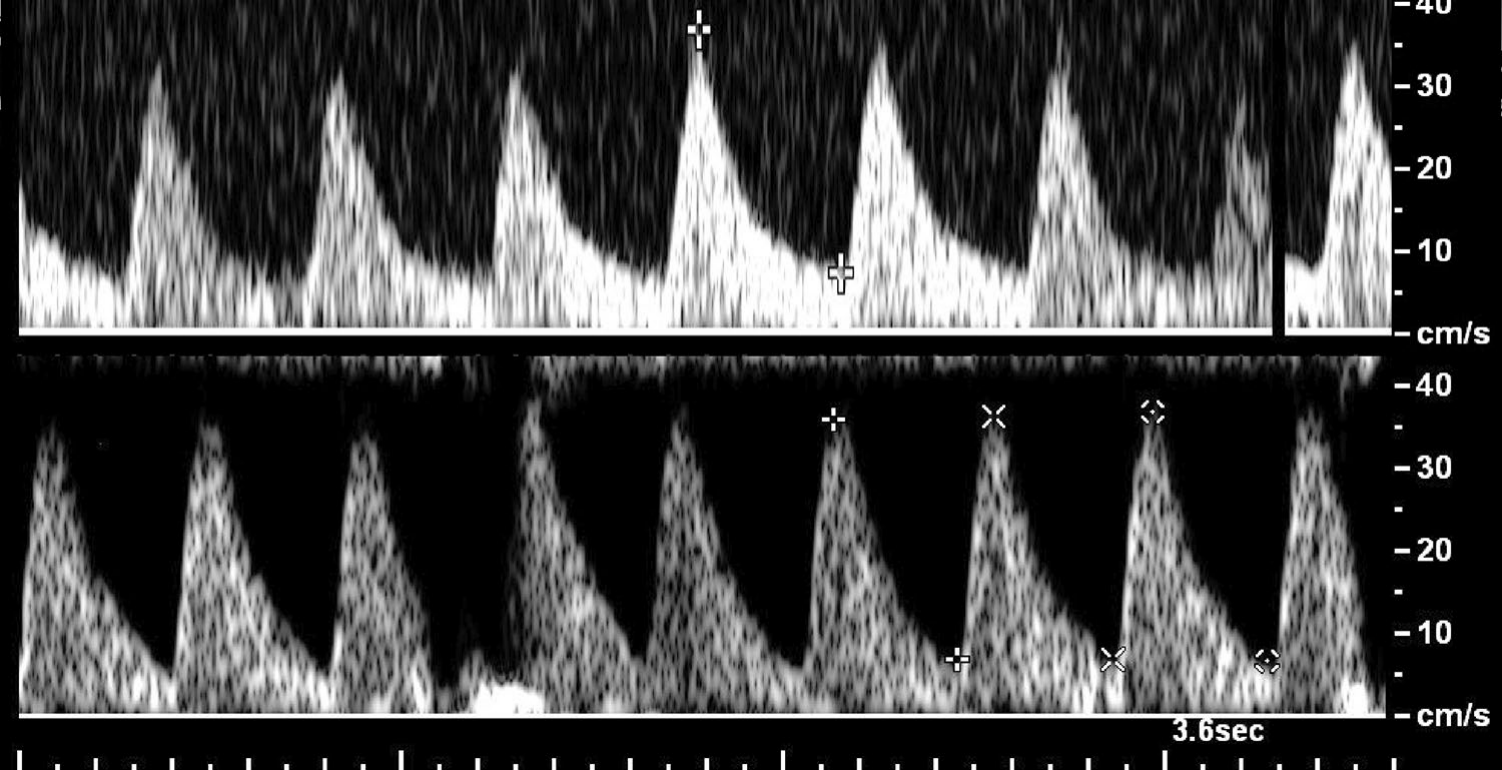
Umbilical artery Doppler flow sonograms from a normally developing pregnancy (top) and from a pregnancy developing intrauterine growth restriction (bottom). The average systolic to diastolic ratio—which is the current Doppler standard for predicting IUGR—in the top image is approximately 5.1, and in the lower image approximately 5.3 (essentially they are indistinguishable), yet preliminary data demonstrate that ML can differentiate between these and other similar UADF images with over 90% accuracy
